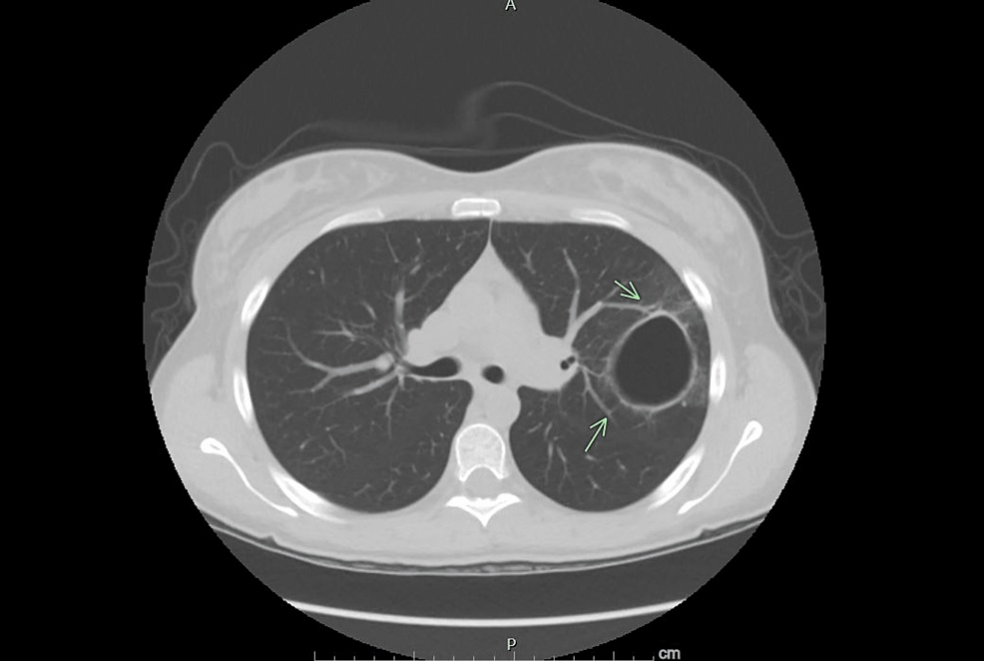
Chest CT done after five weeks of antibiotics showing significant improvement and resolution of air fluid levels
